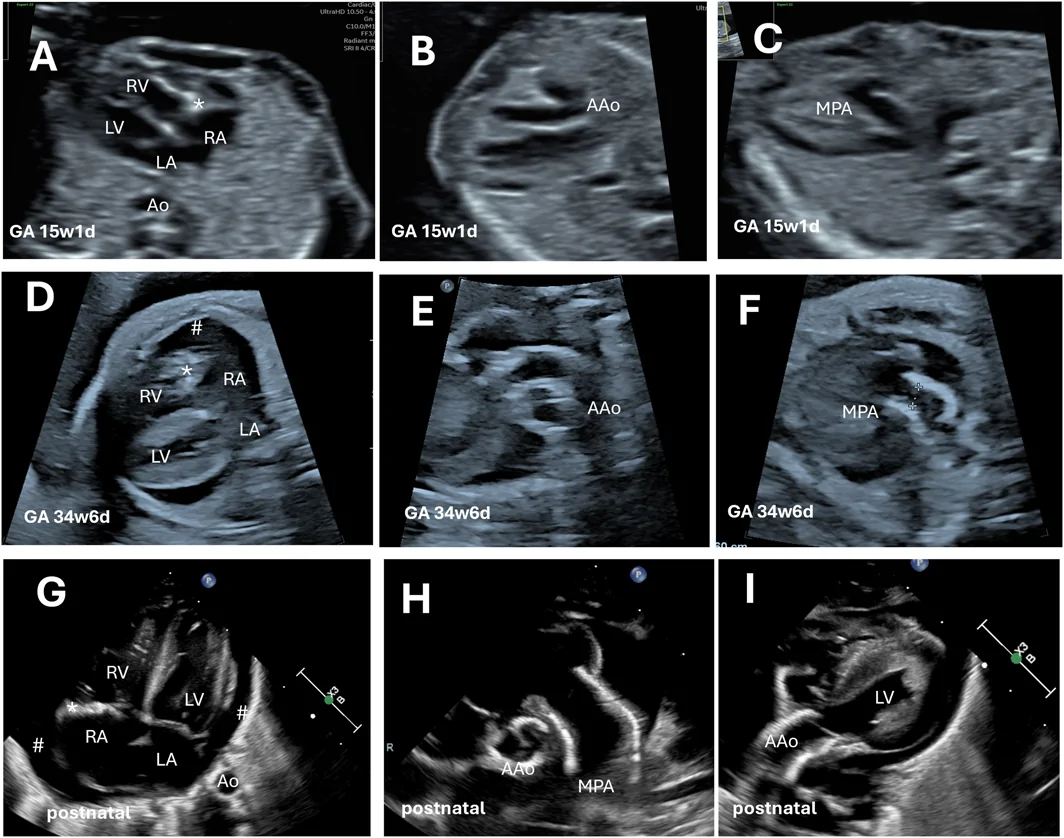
(A, D, G) Four‐chamber views, (B, E, H) left ventricular outflow tract views, and (C, F, I) right ventricular outflow tract views obtained prenatally at 15 + 1 weeks (A–C) and 34 + 6 weeks of gestation (D–F), and postnatally (G–I). Early fetal echocardiography demonstrated increased echogenicity of the tricuspid annulus (*), right ventricular endocardium, ascending aorta (AAo), and main pulmonary artery (MPA), while cardiac anatomy and ventricular function remained preserved. At 34 + 6 weeks, sudden clinical deterioration occurred with severe biventricular myocardial hypertrophy, markedly reduced ventricular contractility, and a large circumferential pericardial effusion (#). Postnatal echocardiography confirmed diffuse cardiovascular calcifications involving the coronary arteries, ascending aorta, and great vessels, associated with persistent myocardial hypertrophy and pericardial effusion. AAo, ascending aorta; Ao, aorta; LA, left atrium; LV, left ventricle; MPA, main pulmonary artery; RA, right atrium; RV, right ventricle.
